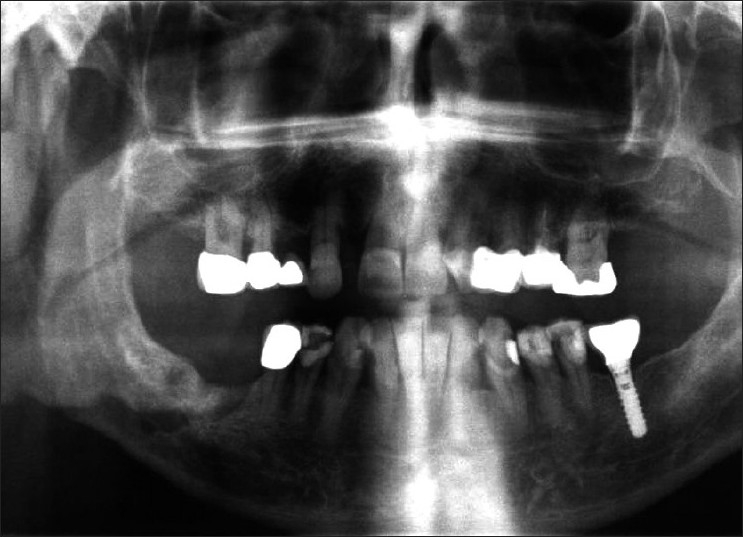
Orthopantomography 6 months postoperatively. The defect at the area of the surgical site as well as the post-extraction socket of 46 are distinguished. Nothing remarkable concerning the bone healing process is observed
